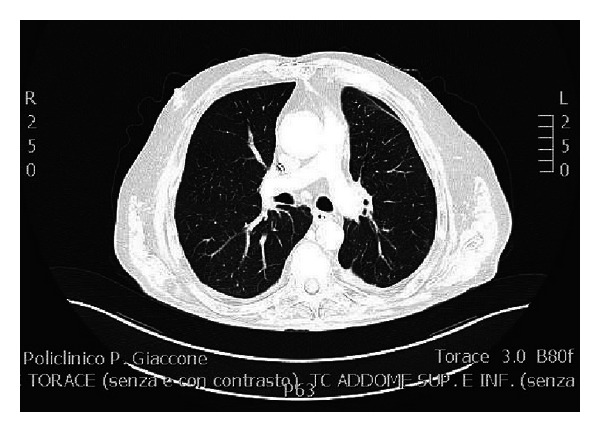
Normal followup CT scan.
